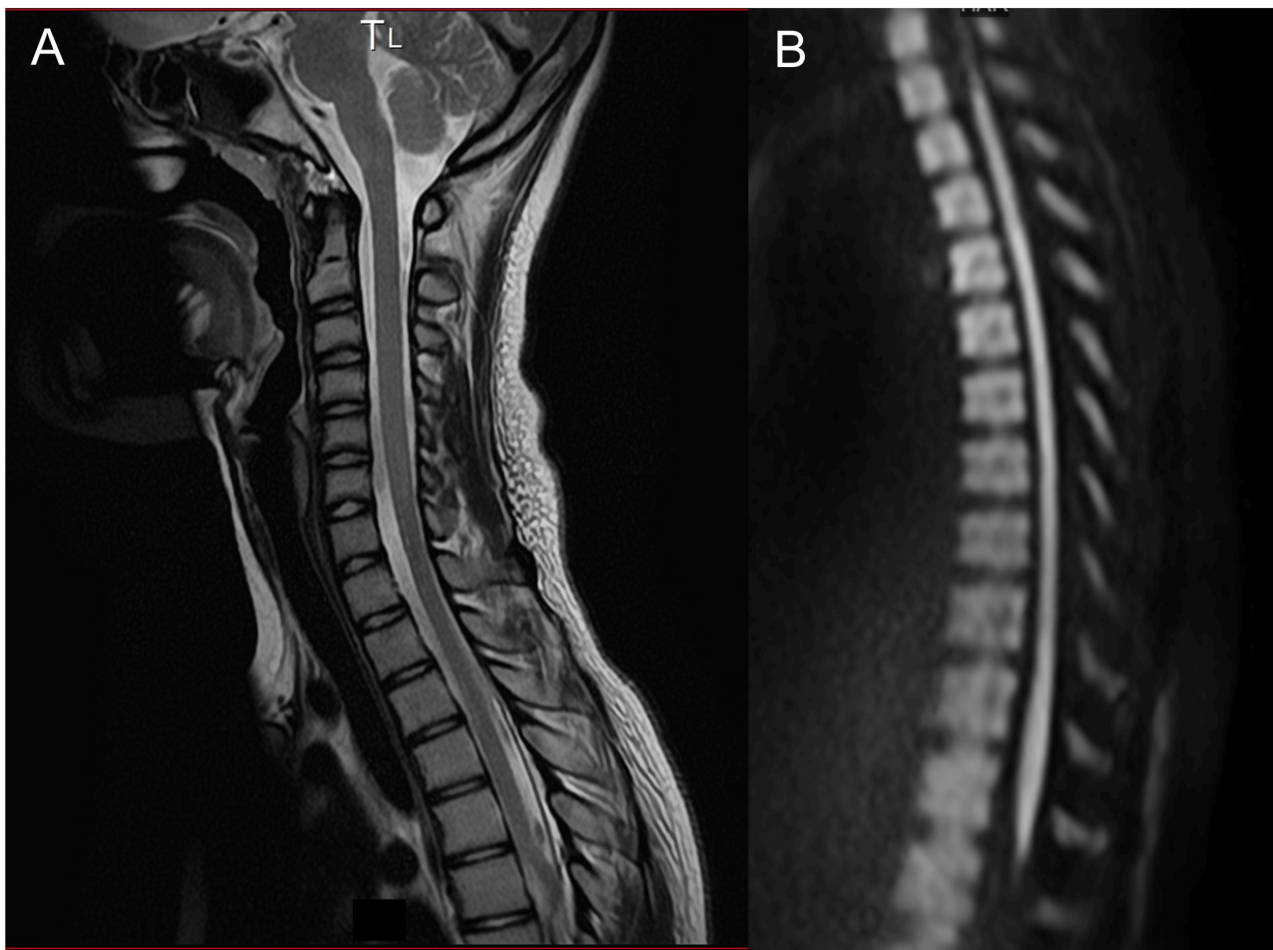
Spinal MRI, including diffusion-weighted imaging, showing no abnormalities on day 1 (A) Sagittal T2-weighted image (cervical-thoracic); (B) sagittal diffusion-weighted image (thoracic-lumbar). No parenchymal abnormalities in the spinal cord were observed.
